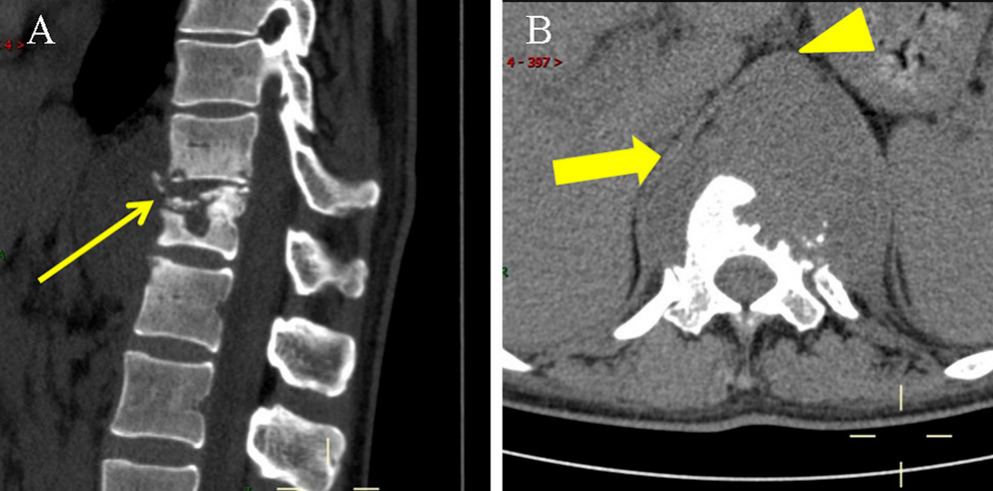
Non-enhanced CT scan on 13 October 2021. (A) Lateral CT scan showed T11-L1 with bone destruction and sequestrums (thin arrow). (B) Axial CT scan showed paravertebral abscess (thick arrow) was indistinguishable from the thoracic aorta (arrowhead).
